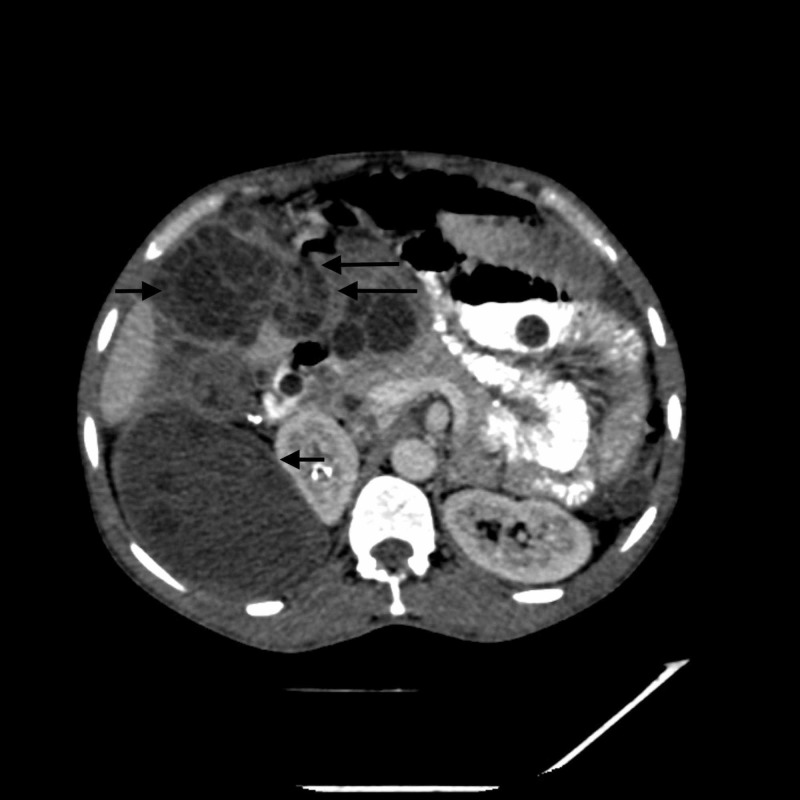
Axial CT image showing communication of peritoneal cyst with distal stomach and proximal duodenum. Long black arrows depict the site of communication. Short black arrows depict peritoneal and retroperitoneal cysts.
